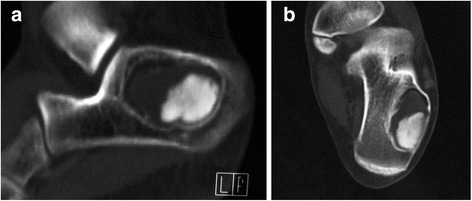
Computed tomography (CT) scans of the calcaneum. a Saggital reconstruction image and (b) coronal reconstruction image show an osteolytic lesion with sclerotic margin and an eccentric focus of matrix calcification adjacent to soft tissue dense with an arc-shaped fat band
